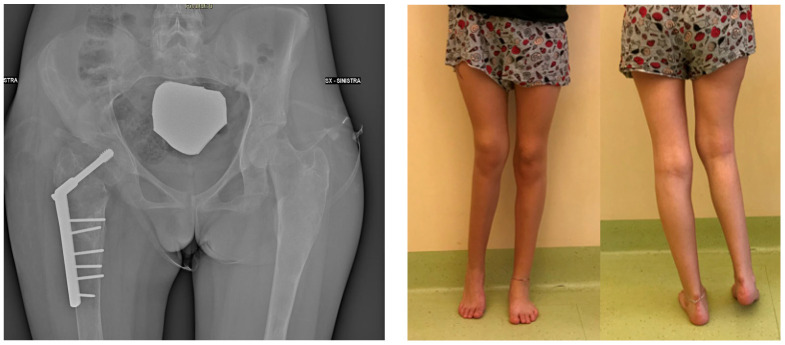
Postoperative X-ray and clinical pictures of the valgus and derotational femoral osteotomy.
